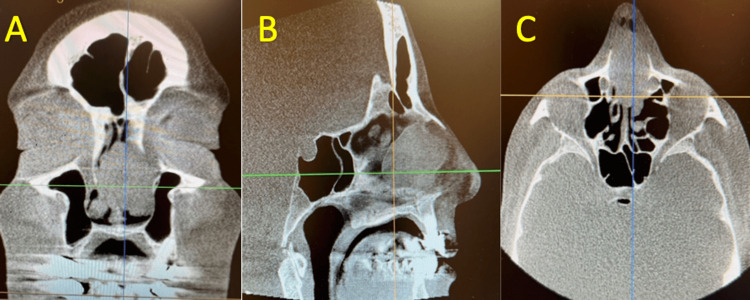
Computerized tomography scan of the nose and sinuses CT imaging reveals a large mass in the right and left nasal septum on coronal (A), sagittal (B), and axial (C) view, respectively. Coronal view (A) depicts a greater left sided nasal septum involvement of the mass compared to the right nasal septum.
